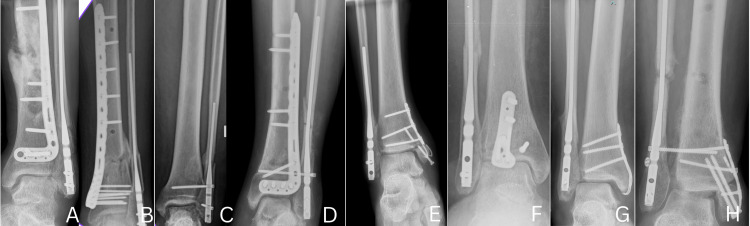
Postoperative radiographs at four to six months demonstrating maintained reduction and radiographic union except for one case of asymptomatic nonunion following fibular nail fixation This figure is original and derived from data collected in the present study. The alphabetical identifiers (e.g., A, B, C, etc.) used in the figure are arbitrary and were created solely for the purpose of referencing specific cases within this article. These identifiers do not correspond to any patient-identifying information.
